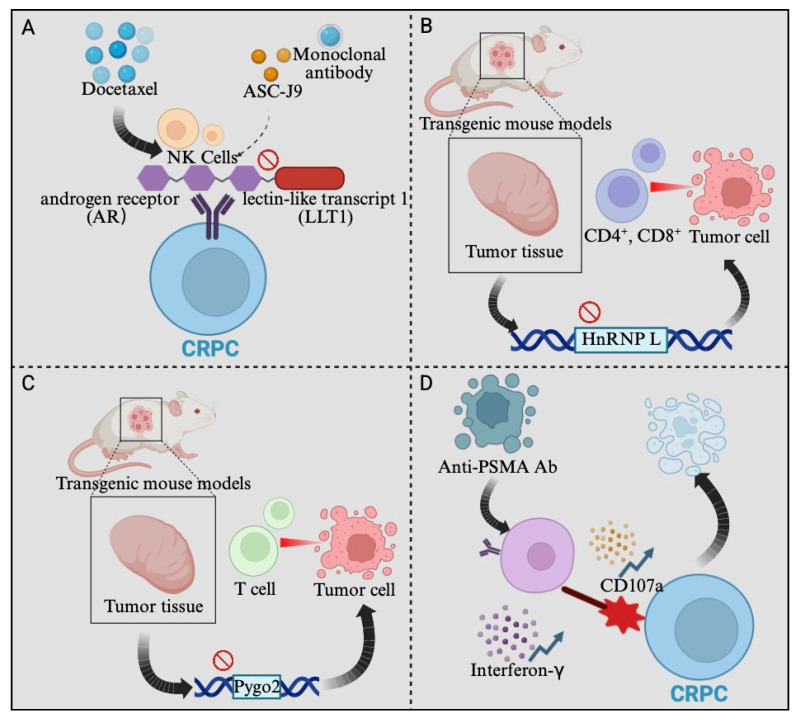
(A) Targeting AR with ASC-J9 or blocking LL1 by anti-human LLT1 monoclonal antibody. (B) Knocking out HnRNP L inhibits the development of tumors. (C) Knocking out Pygo2 inhibits the development of tumors. (D) Anti-PSMA antibody enhances NK cell-mediated cytotoxicity against CRPC cells, evidenced by upregulation of CD107a and increased interferon-γ secretion. Created in BioRender. Xia, X. (2026). BioRender.com/42tex64 (accessed on 10 January 2026).
